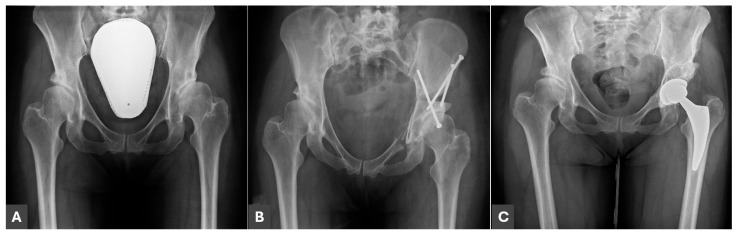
A 45-year-old female patient with residual DDH. (A) AP radiograph before PAO; (B) AP radiograph after PAO of the left hip; (C) AP radiograph after screw removal and THA subsequently to PAO.
